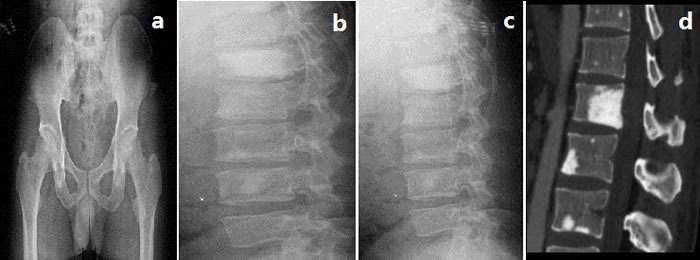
Skeletal radiographic survey and computed tomography (CT)
